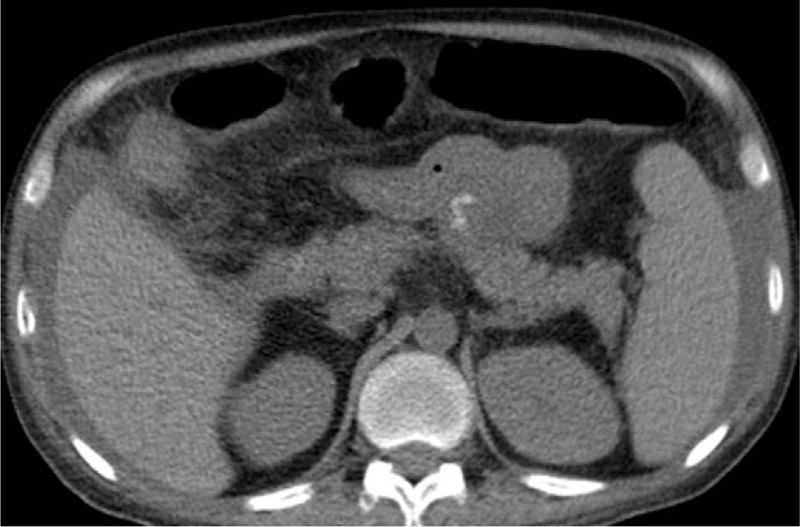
Plain abdominal CT scan showing splenomegaly and ascites.
